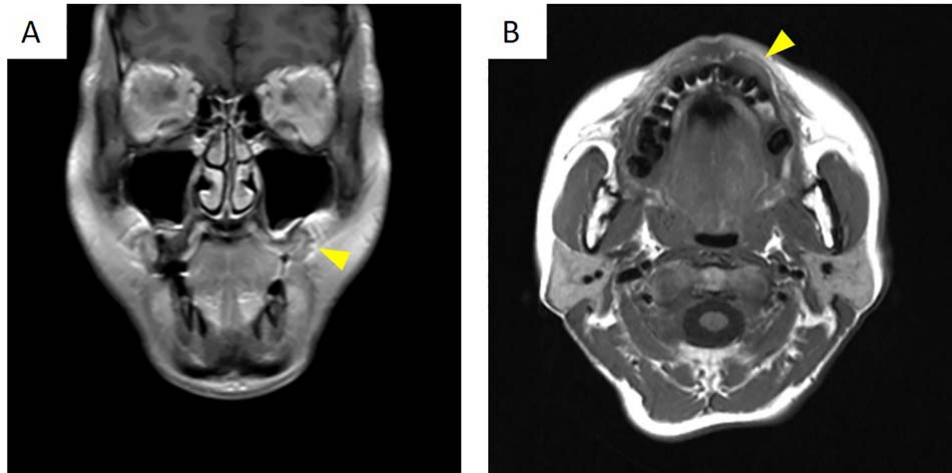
On magnetic resonance imaging, contrast-enhanced T1-weighted images showed a row signal. (A) Frontal cross-section. (B) Horizontal cross-section. The arrowheads indicate the tumor.
